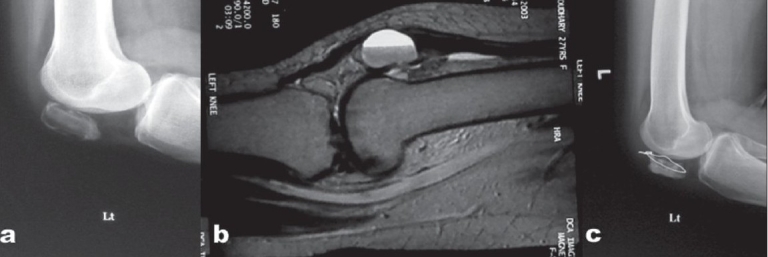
X-ray of left knee (lateral view) showing stage 3 ABC in left patella (a) T_2_ weighted saggital MRI of left knee showing fluid level in the cystic patella (b) Two-year follow-up X-ray showing well formed patella but with central lucency (c)
